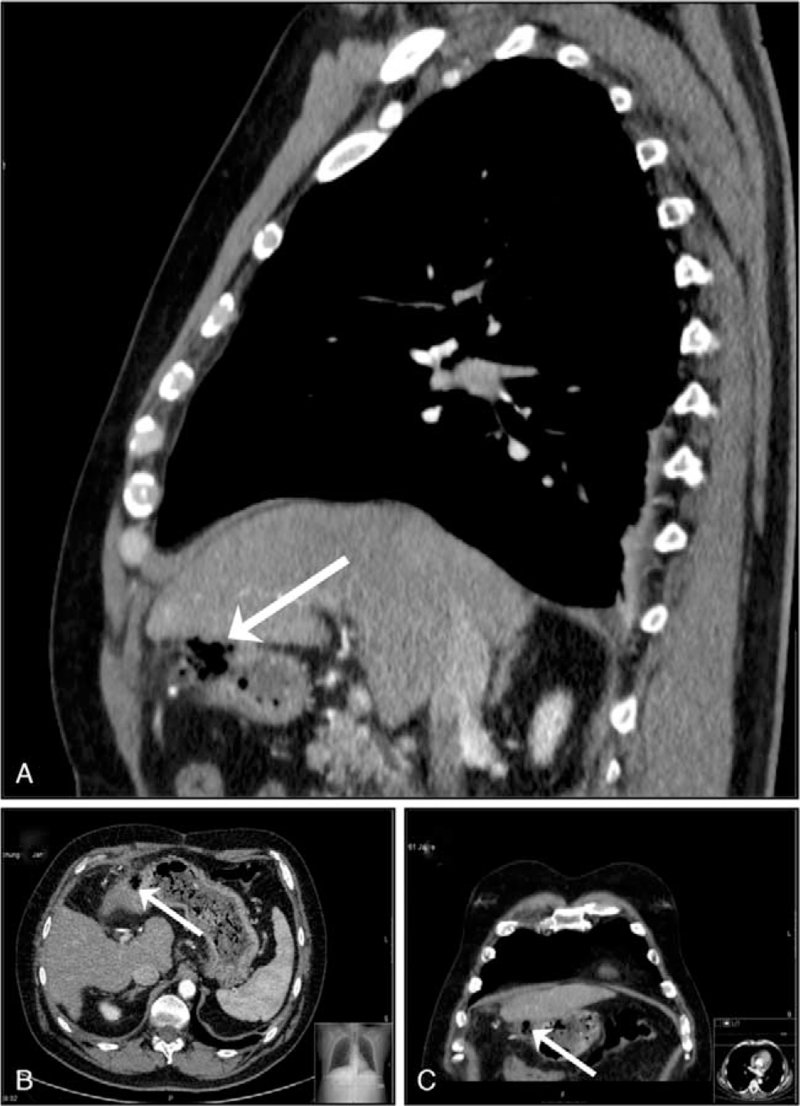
Contrast enhanced computed tomography 2 weeks after percutaneous microwave ablation of hepatocellular carcinoma nodules in segments III and VIII. Sagittal and horizontal images reveal a defect of the gastric antrum wall in direct vicinity a necrotic ablation area in segment III of the liver (A, B, arrows). Of interest, frontal images show that the adjacent liver covers the defect (C, arrow).
